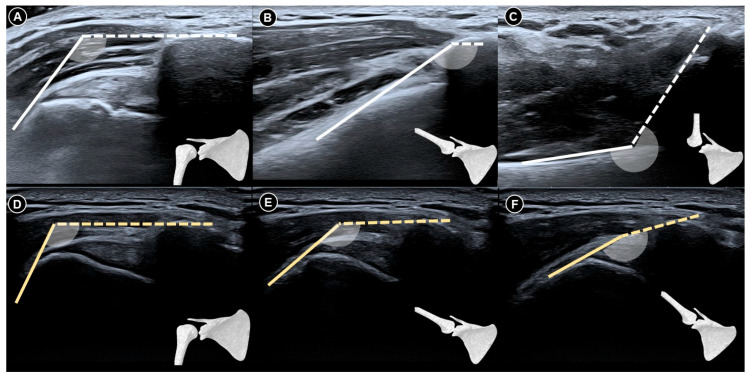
Dynamic ultrasound images of a normal shoulder captured at neutral resting position (A), during abduction beyond 90 degrees (B), and at full elevation to 180 degrees (C). Corresponding images of a shoulder with adhesive capsulitis at rest (D), abduction past 90 degrees (E), and maximal abduction (F). The figure was redrawn by the authors with reference to Chang et al. [35].
